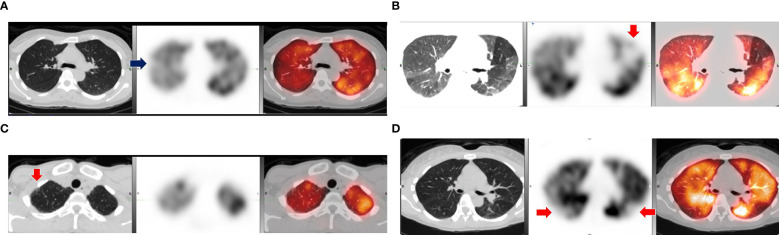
Comparison of abnormal lung SPECT-CT results among cases and controls with positive findings. (A) Small wedge-shaped perfusion defect in lateral segment of right upper lobe (arrow). (B) Medium size defect in anterior left upper lobe (arrow) and evidence of air trapping. (C) Small airway disease in anterior right upper lobe (arrow) without perfusion defect. (D) Control with multiple subsegmental perfusion defects in bilateral lower lobes (arrows). Notes: Scans of positive cases (A–C) and a positive control (D) with CT scan (left), SPECT perfusion scan (middle), and fused SPECT-CT (right).
